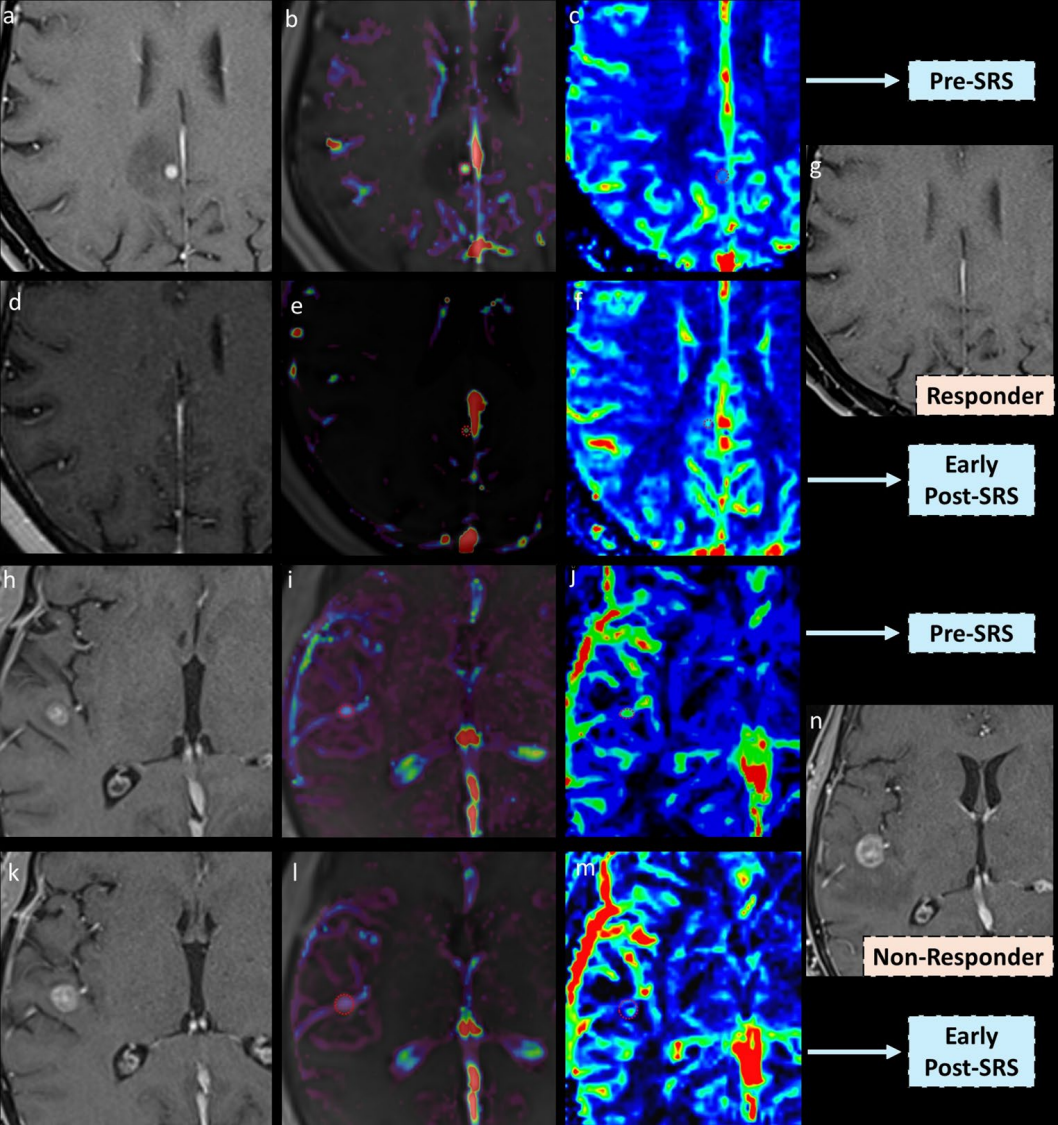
Representative cases of stereotactic radiosurgery (SRS) responder and non-responder brain metastases (BMs) from lung carcinoma, demonstrating multiparametric MRI findings at pre- and early post-SRS timepoints. (a) In the responder case, a 66-year-old patient with lung adenocarcinoma had a solid metastasis located in the right deep parietal white matter (precuneus). (b) Pre-SRS K-trans was measured at 0.058 min⁻¹, and (c) the normalized cerebral blood volume (nCBV) was 3.4 with the same region of interest (ROI). (d) Four weeks after SRS, there was a marked reduction in lesion size and perilesional edema. (e) The early post-SRS K-trans decreased to 0.042 min⁻¹, corresponding to a 28% reduction. (f) nCBV of BMs decreased to 2.9 at the early post-SRS stage. (g) At the 28-week midterm follow-up, the lesion had completely resolved with regressed surrounding edema consistent with a complete treatment response. (h) In the non-responder case, a 55-year-old patient with small cell lung carcinoma had a solid metastasis in the right superior temporal gyrus. (i) Pre-SRS K-trans of the BMs was 0.121 min⁻¹, and (j) the corresponding nCBV was 3.9 with the same ROI. (k) Early post-SRS imaging showed a slight increase in lesion size, (l) with a persistently high K-trans of 0.125 min⁻¹, and (m) a modest reduction in nCBV to 3.2. (n) At the 24-week midterm follow-up, the lesion is enlarged further with prominent surrounding edema, necessitating an additional SRS session. In this non-responder case, persistently high K-trans despite a mild nCBV decrease suggested treatment resistance and poor response to SRS
